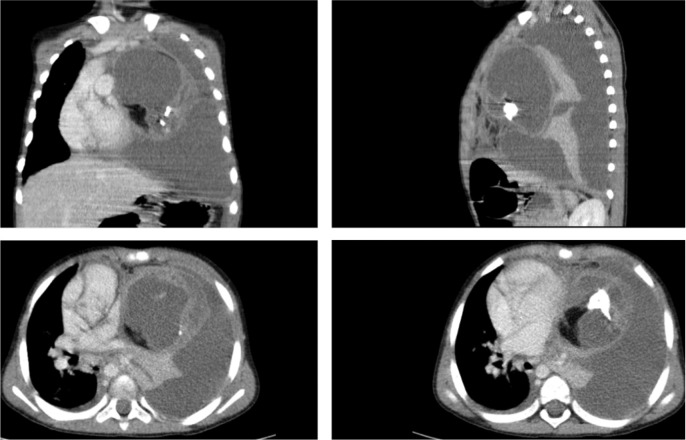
Multiplanar contrast‑enhanced CT scan.
